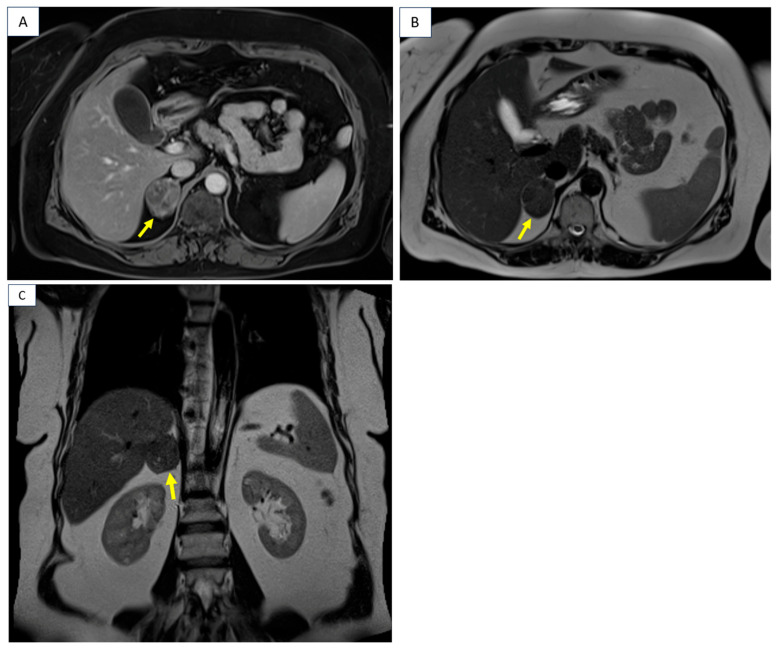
MRI confirmed similar features of the adrenal tumors. [4. Contrast-enhanced MRI scan: right adrenal tumor of 4.2 by 3.3 by 4.5 cm with intracellular lipid content and no restricted diffusion on diffusion-weighted imaging (DWI) (yellow arrow); (A) T1 VIBE Dixon, axial plane; (B) T2 HASTE, axial plane; (C) T2 HASTE, coronal plane].
